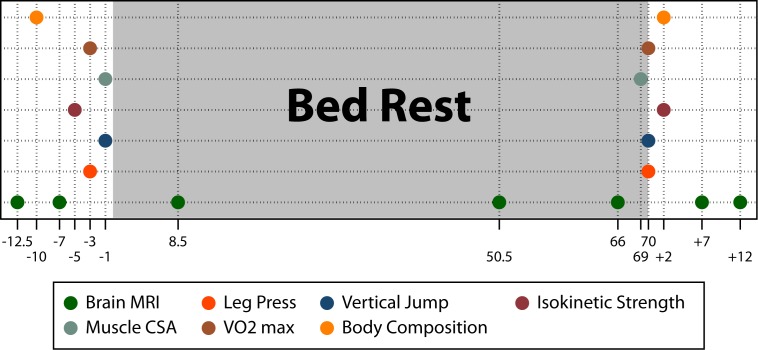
Time line of data collection of brain MRI and physical fitness measures. The x-axis indicates the number of days relative to the HDBR intervention (e.g., -12.5 = 12.5 days pre-HDBR; 8.5 = days in HDBR; and +2 = 2 days post-HDBR). Brain MRI = structural T1-weighted MRI, diffusion weighted imaging, and resting state functional connectivity MRI; Leg Press = lower body strength (isometric, isokinetic, total work); Vertical Jump = vertical jump (height and Watt) Isokinetic Strength = isokinetic knee and ankle extension and flexion strength; Muscle CSA = muscle cross-sectional area (soleus, quadriceps, hamstrings); VO2 max = rate of oxygen consumption during peak performance; Body Composition = fat mass and fat free mass.
